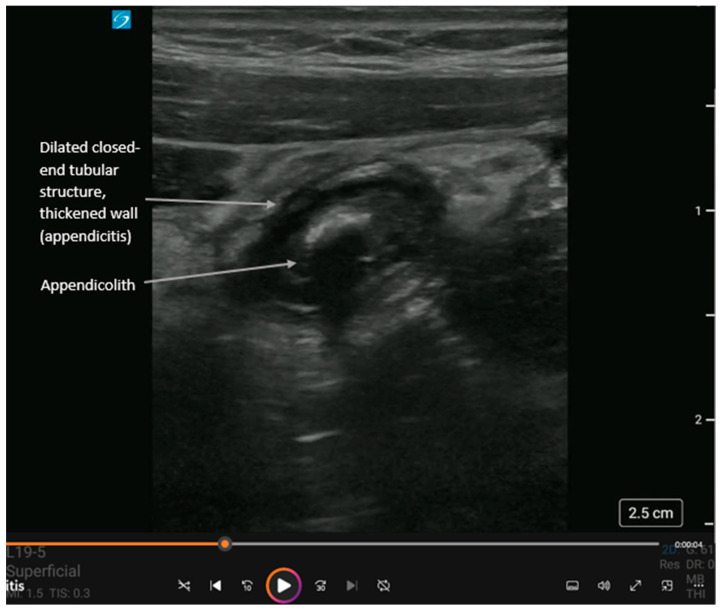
Short-axis view of acute appendicitis with an appendicolith and dynamic findings in Supplementary Video S4. The appendix is a closed-ended tubular structure usually found in the right lower quadrant of the abdomen. Landmarks for locating the appendix are the psoas muscle laterally and inferiorly, the external iliac vessels medially and inferiorly, and the internal oblique and transversus abdominis muscles anteriorly. In cases of acute appendicitis, the appendix diameter is dilated > 5 mm and non-compressible. Like with diverticulitis, ultrasound shows (i) thickened bowel wall > 3 mm, (ii) hyperemic bowel wall, and (iii) hyperechoic surrounding fat. An appendicolith (hyperechoic circular structure with posterior shadowing) or debris may be visualized within the bowel lumen. Also, complications can include extra-luminal free fluid (anechoic adjacent to the appendix), an abscess (heterogeneous collection adjacent to the appendix), or perforation (disruption of the appendiceal wall with either free fluid or abscess). While operator-dependent, recent meta-analyses report pooled sensitivity and specificity for POCUS diagnosis of appendicitis to be approximately 81% and 87%, respectively, making it a highly effective rule-in tool [19,20,21,22].
